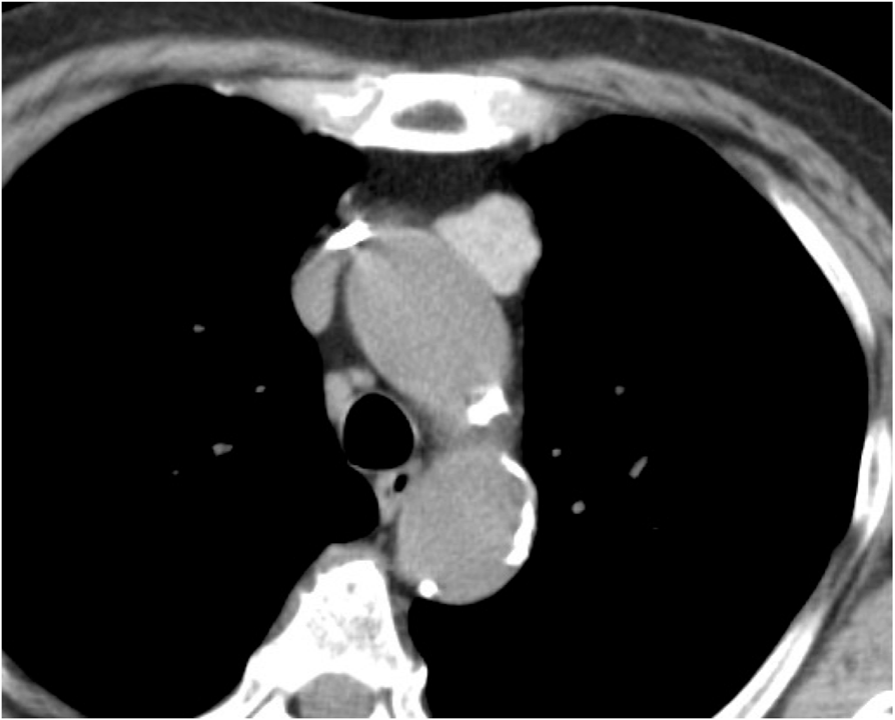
Preoperative imaging findings. Non-enhanced computed tomography (CT) scan. A solitary homogeneous anterior mediastinal tumor (2.0 cm in size) showed high radiodensity (CT value: 91.9 Hounsfield units)
